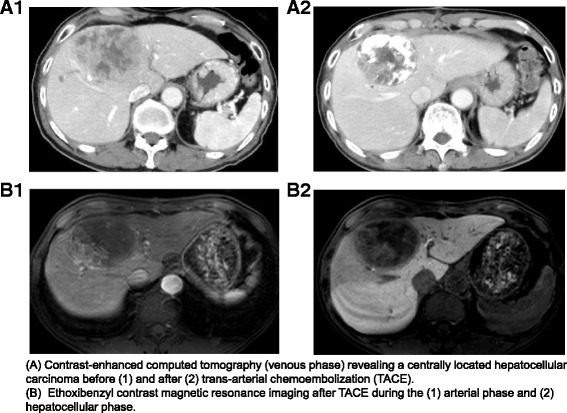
Hepatocellular carcinoma. a Contrast-enhanced computed tomography (venous phase) revealing a centrally located hepatocellular carcinoma before (1) and after (2) trans-arterial chemoembolization (TACE). b Ethoxibenzyl contrast magnetic resonance imaging after TACE during the (1) arterial phase and (2) hepatocellular phase
